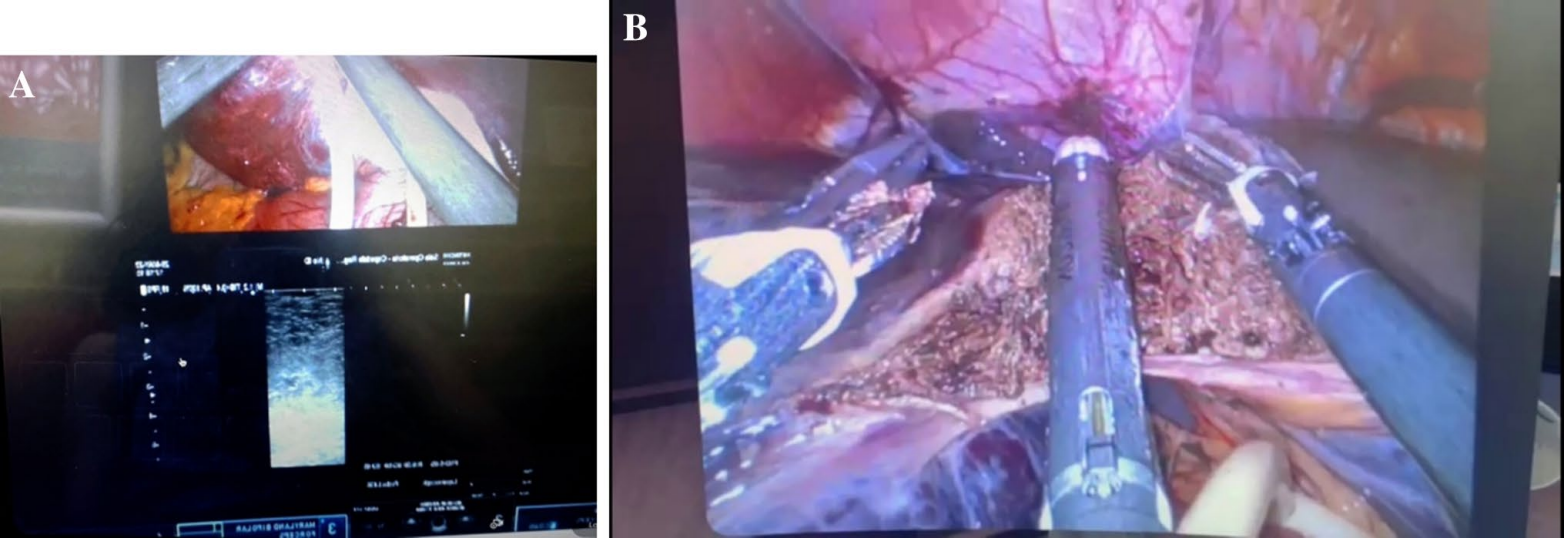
A Liver visualization with ultrasound robotic probe. B Final liver dissection
